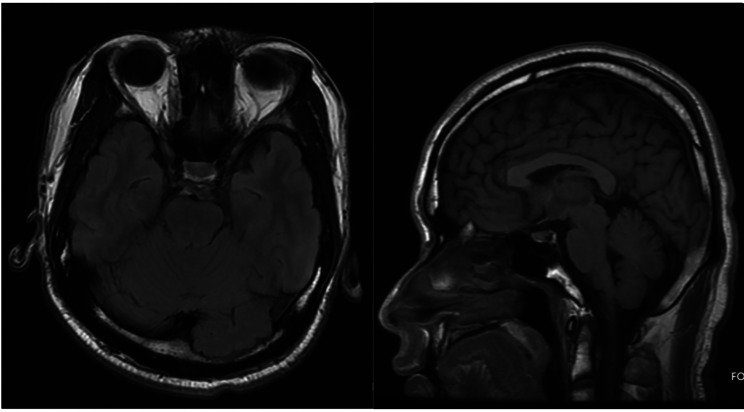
Brain MRI of the patient. Brain MRI showed no obvious abnormalities
